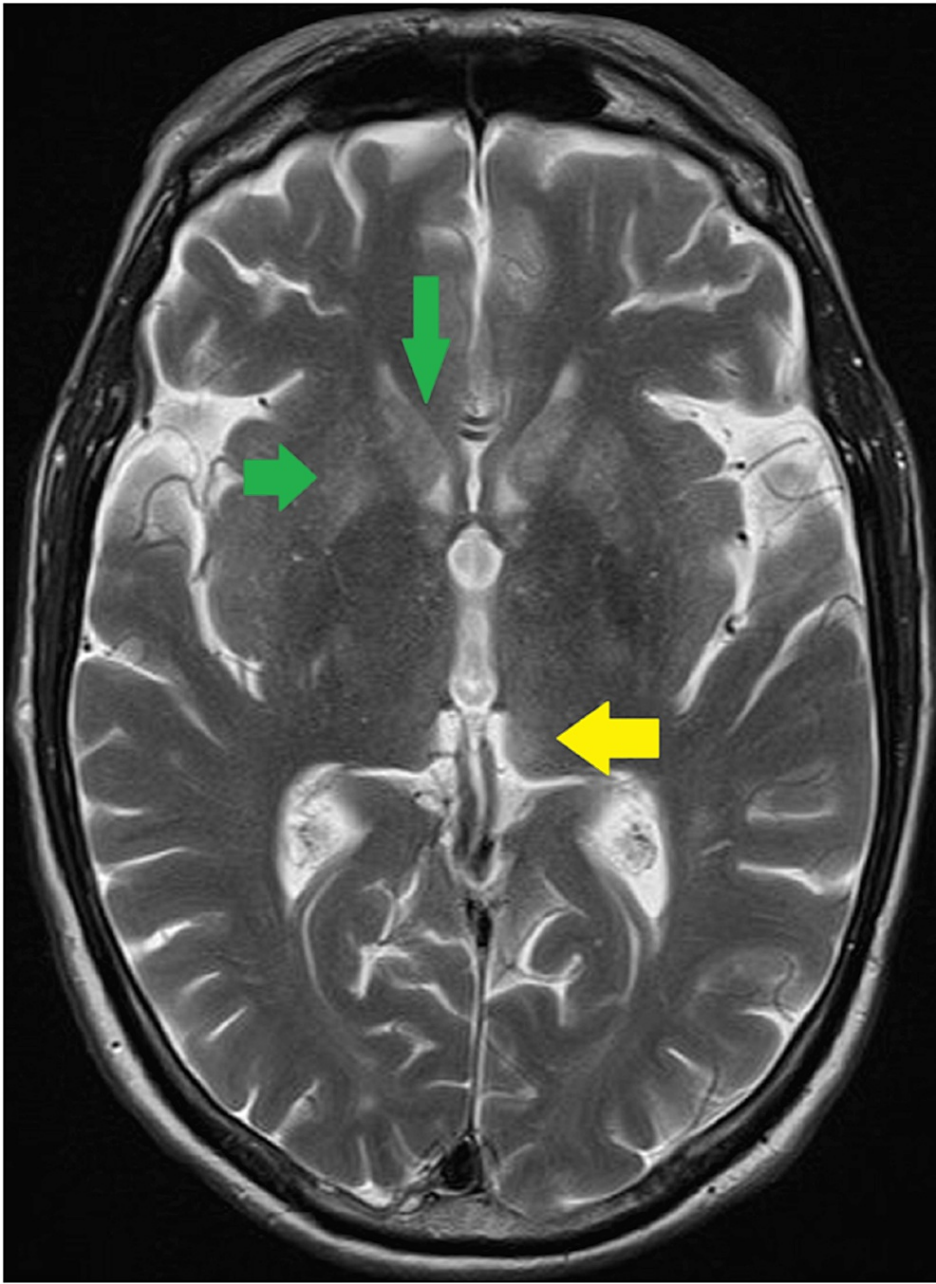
MRI Brain T2-weighted axial image showing hyperintense signals in the head of bilateral caudate nuclei, putamen (green arrows), and dorsomedially in the left thalamus (yellow arrow).
